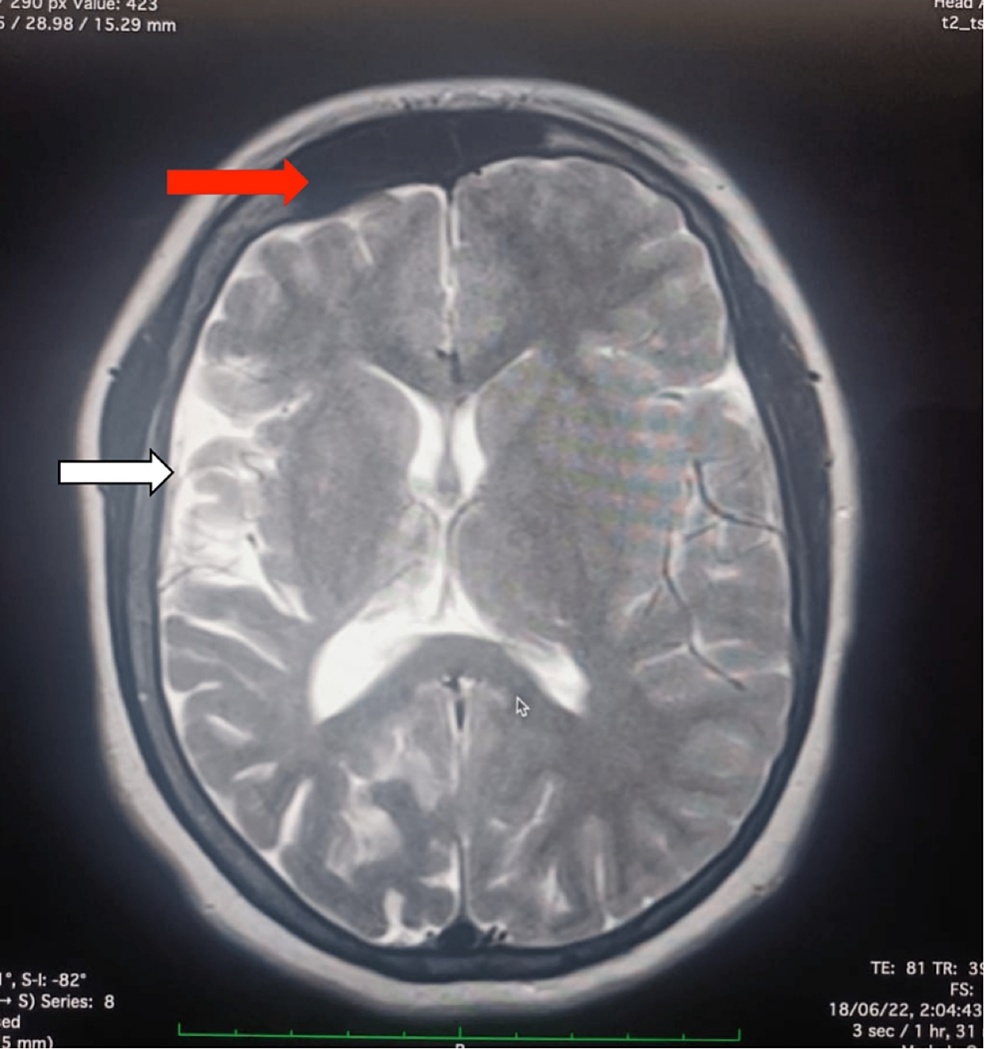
Axial T2-weighted MRI of the brain demonstrates hemiatrophy changes in the right cerebral hemisphere (white arrow) and hyperpneumatization of the frontal sinus (red arrow). MRI: magnetic resonance imaging
